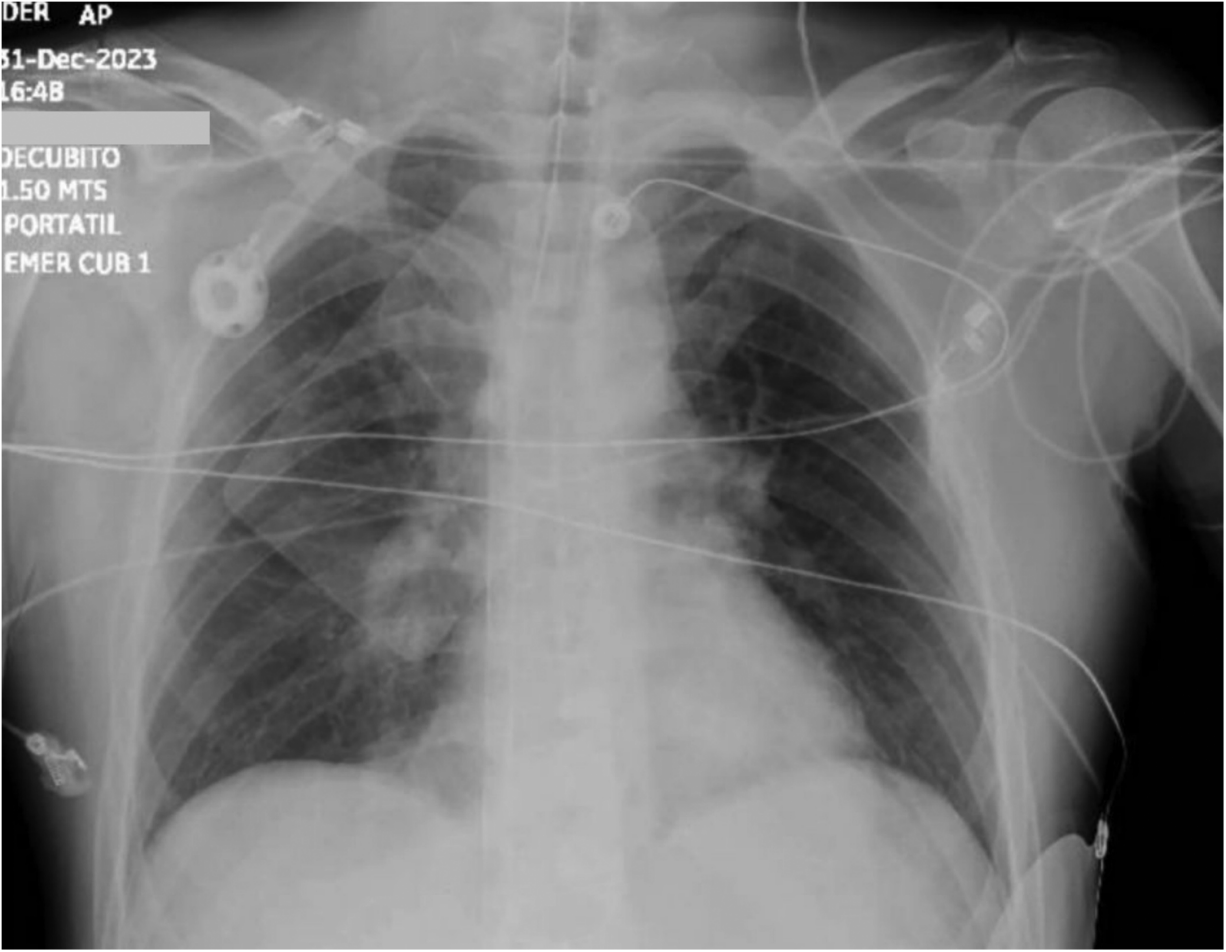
Chest x-ray shows right pulmonary artery amputation and bilateral Westermark sign.
